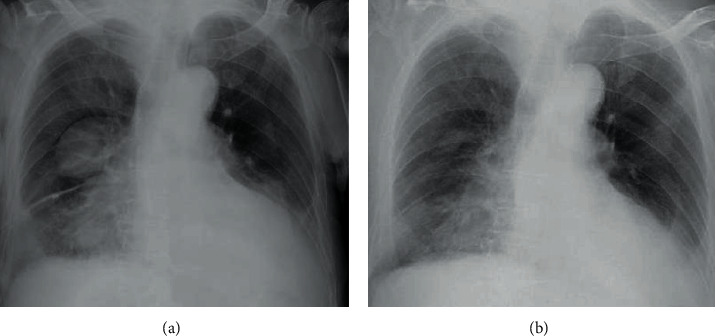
Chest radiograph. (a) Chest drainage removal date (June 28, 2021). (b) End date of 3 courses of triple therapy (September 9, 2021).
